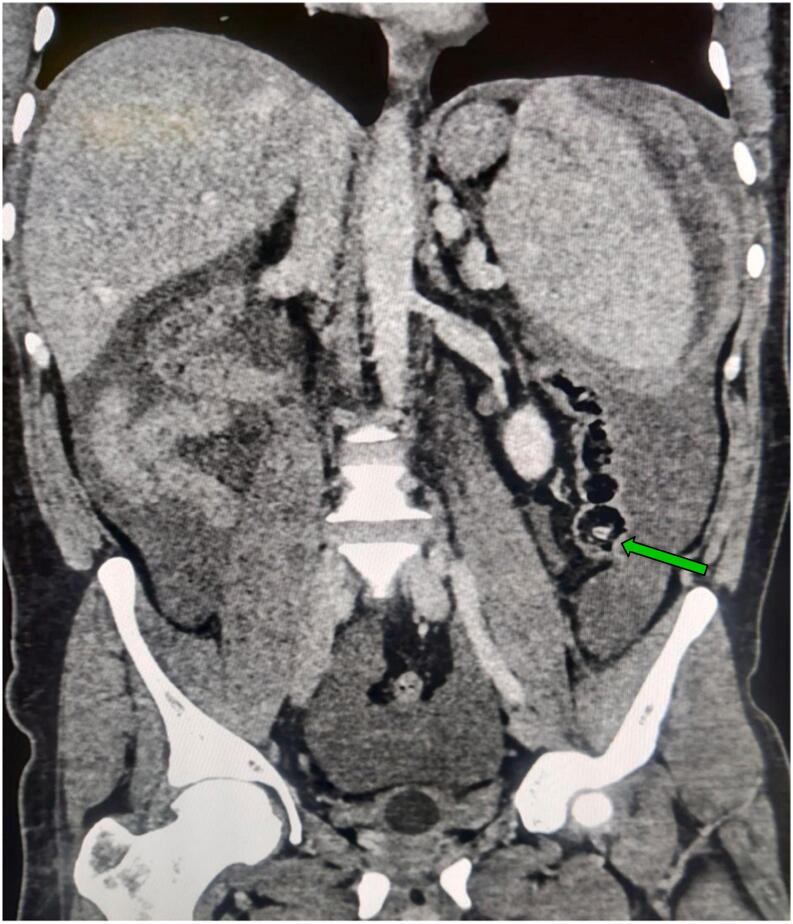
Contrast-enhanced computed tomography (CECT) of the abdomen and pelvis, venous phase, coronal section showing cecum in the left lower quadrant.
